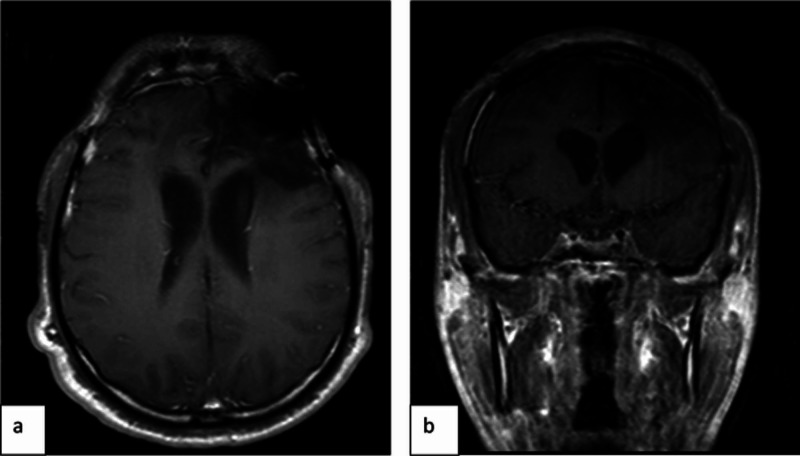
Postoperative MRI brain Post-contrast T1 fat saturation images in axial (a) and coronal (b) planes show edema at the surgical bed with no residual enhancing tumor. MRI: magnetic resonance imaging
